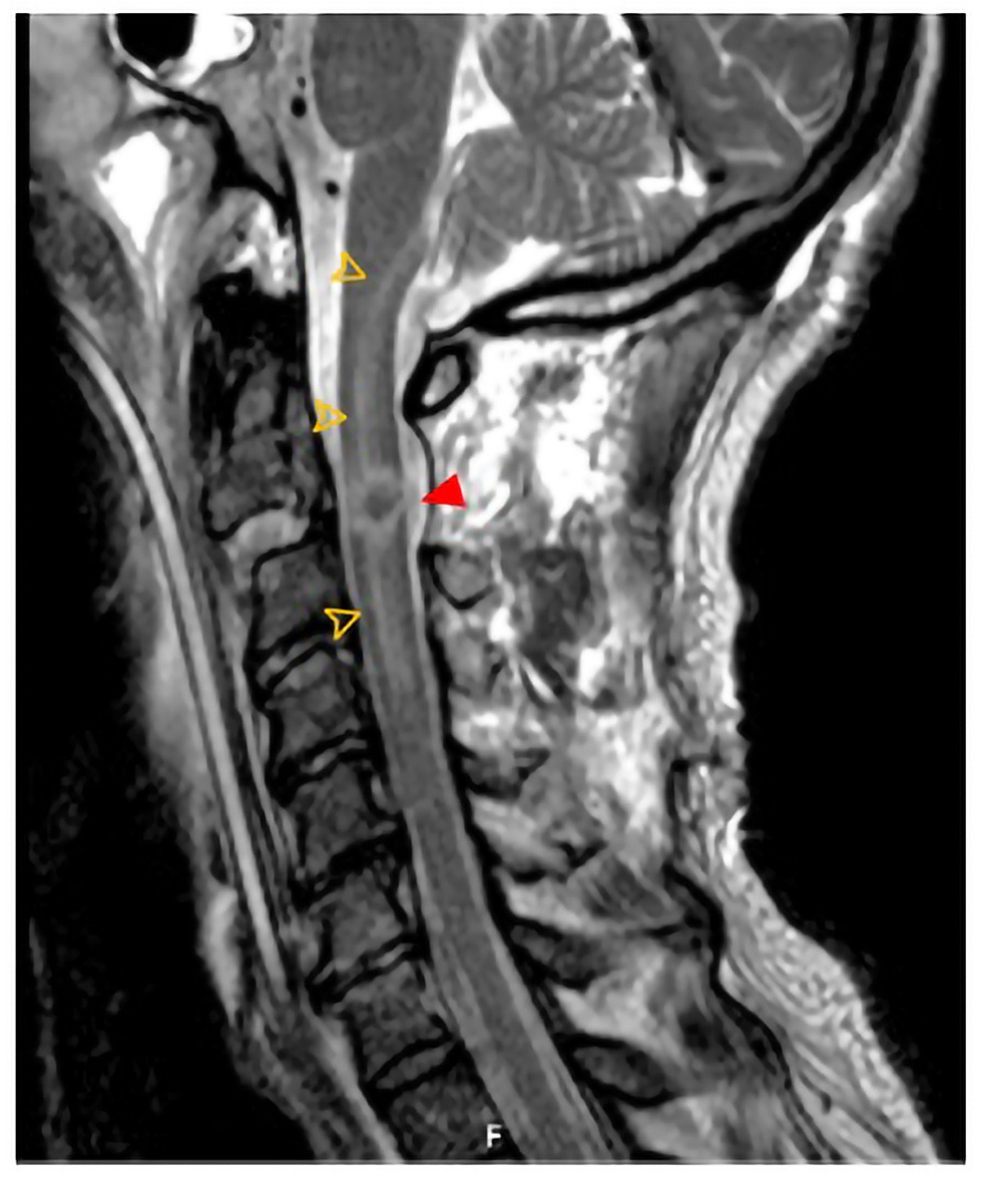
Postoperative cervical MRI scan (sagittal) The scan shows effective spinal cord decompression but important spinal cord contusion at C2-C3 (red arrowhead) due to important adjacent swelling until medulla (yellow arrows). MRI, magnetic resonance imaging
